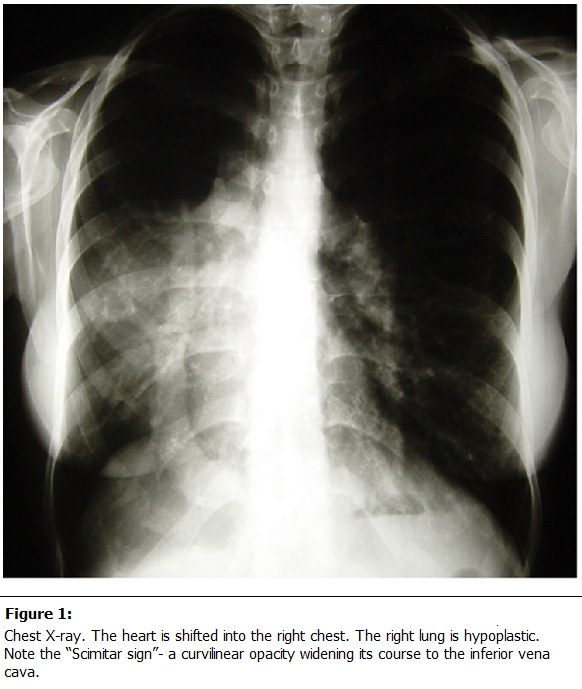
Chest X-ray. The heart is shifted into the right chest. The right lung is hypoplastic. Note the “Scimitar sign”- a curvilinear opacity widening its course to the inferior vena cava.
